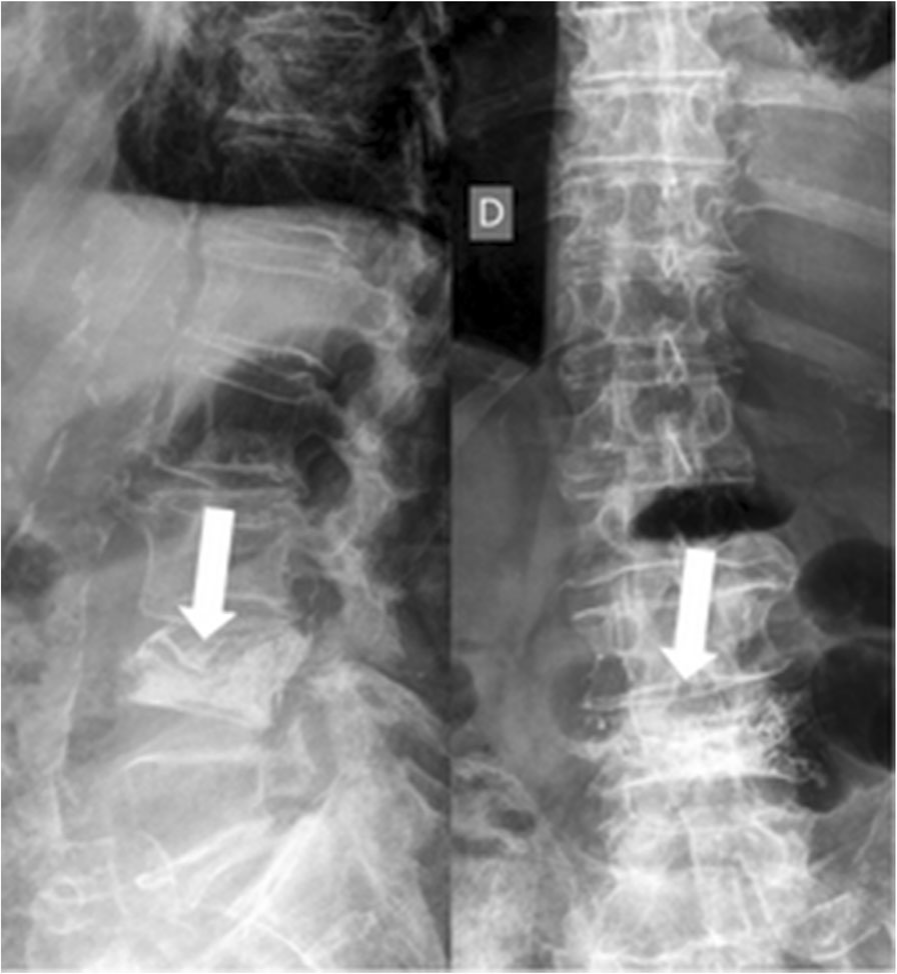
Same case as shown in Figs. 1 and 2. Lumbosacral spine x-ray examination performed one month after the elastoplasty procedure demonstrating the good filling of L4 vertebral body (arrows). No evidence of new vertebral fractures is seen
